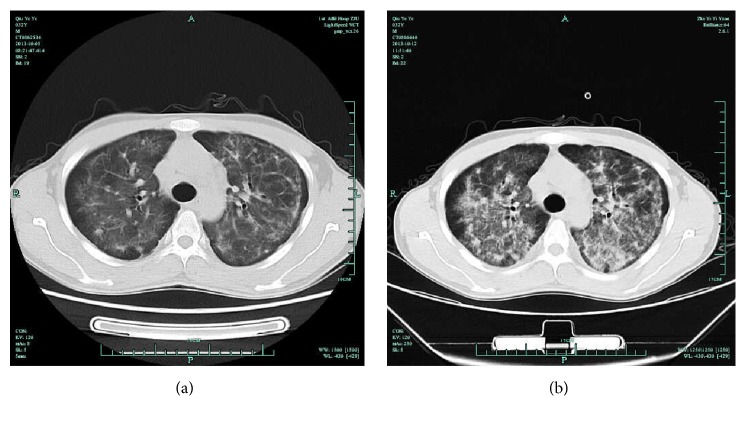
The chest images of computed tomography (CT) scan in the AIDS PCP patient. (a) shows bilateral, symmetric, interstitial, or granular opacities in typical case. (b) shows the opacities are perihilar and diffuse in severe case.
